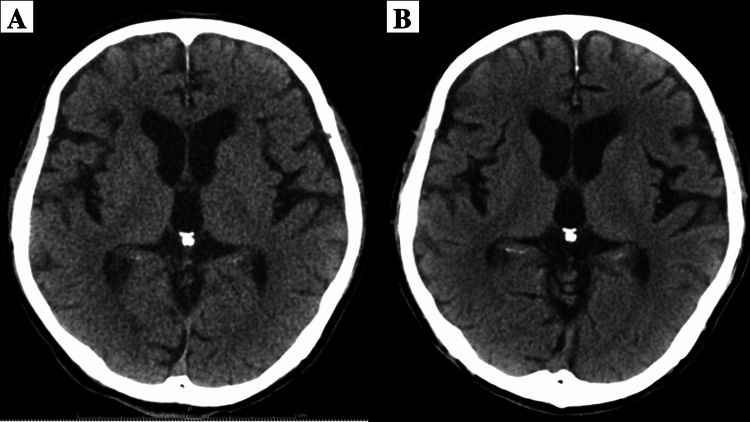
Computed tomography images of the head (A) hospital day zero and (B) one year previously, showing a mild loss of gray-white matter discrimination (GWMD) at the basal ganglia compared with a CT image taken one year earlier
